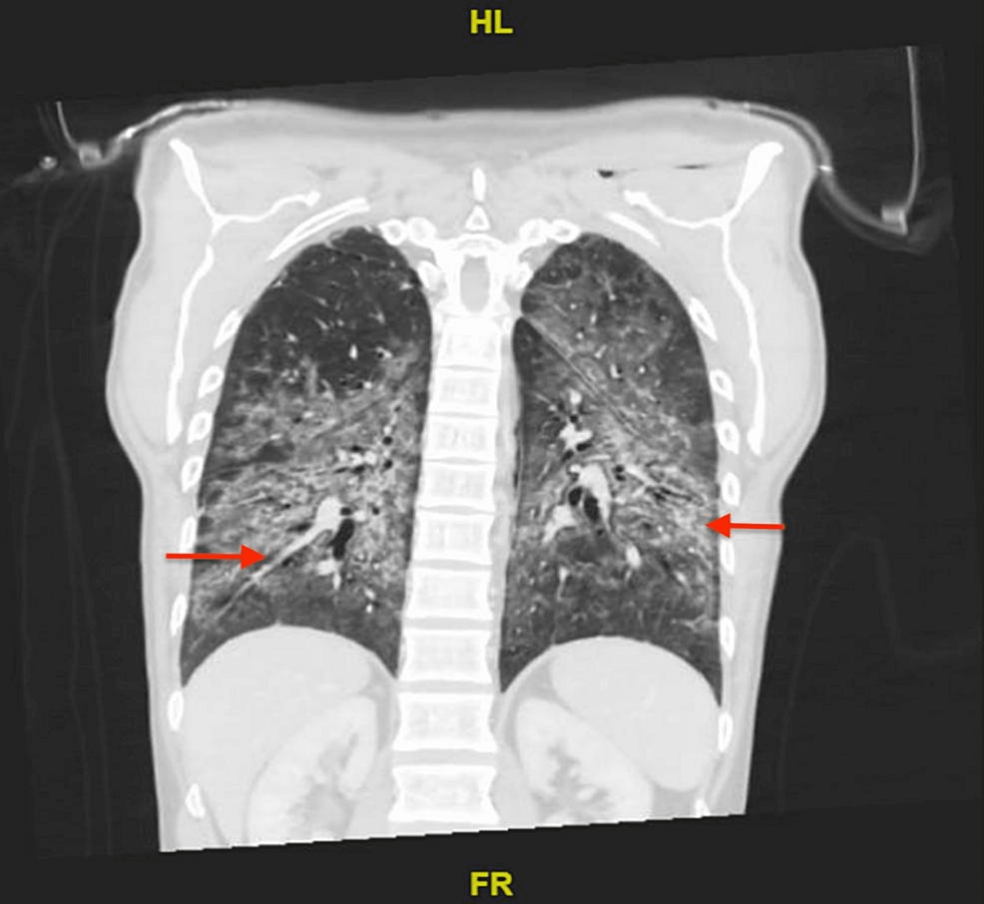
Chest CT with contrast Bilateral bronchiectasis affecting the lower lobes. There are patchy bilateral dependent ground glass opacities with associated tree-in-bud nodules along with interlobular septal thickening and traction bronchiectasis.
